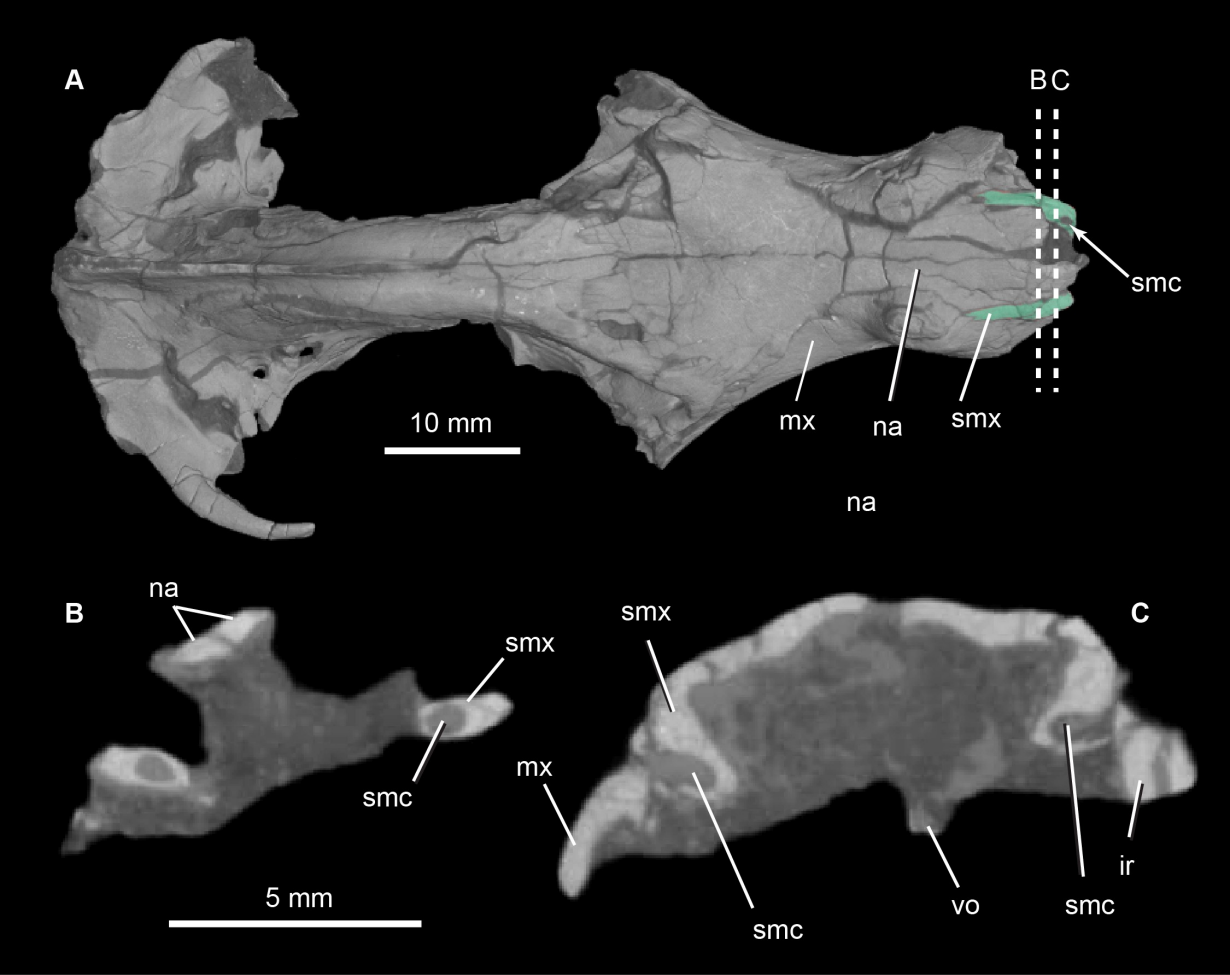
Pseudotherium argentinus*, the septomaxillary canal. (A) 3D volumetric rendering of dorsal view of skull showing septomaxillae in aqua tint (A), and dashed lines that indicate positions of cross sectional CT image slices (B) and (C). Abbreviations: ir, broken incisor root; mx, maxilla; na, nasal; smc, septomaxillary canal; smx, septomaxilla, vo, vomer.
